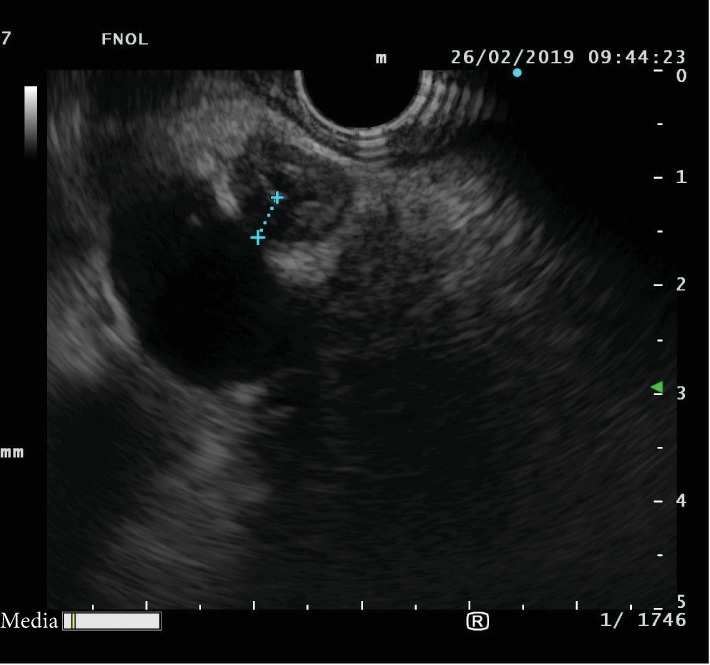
Linear endosonography view of a distal cholangiocarcinoma.
